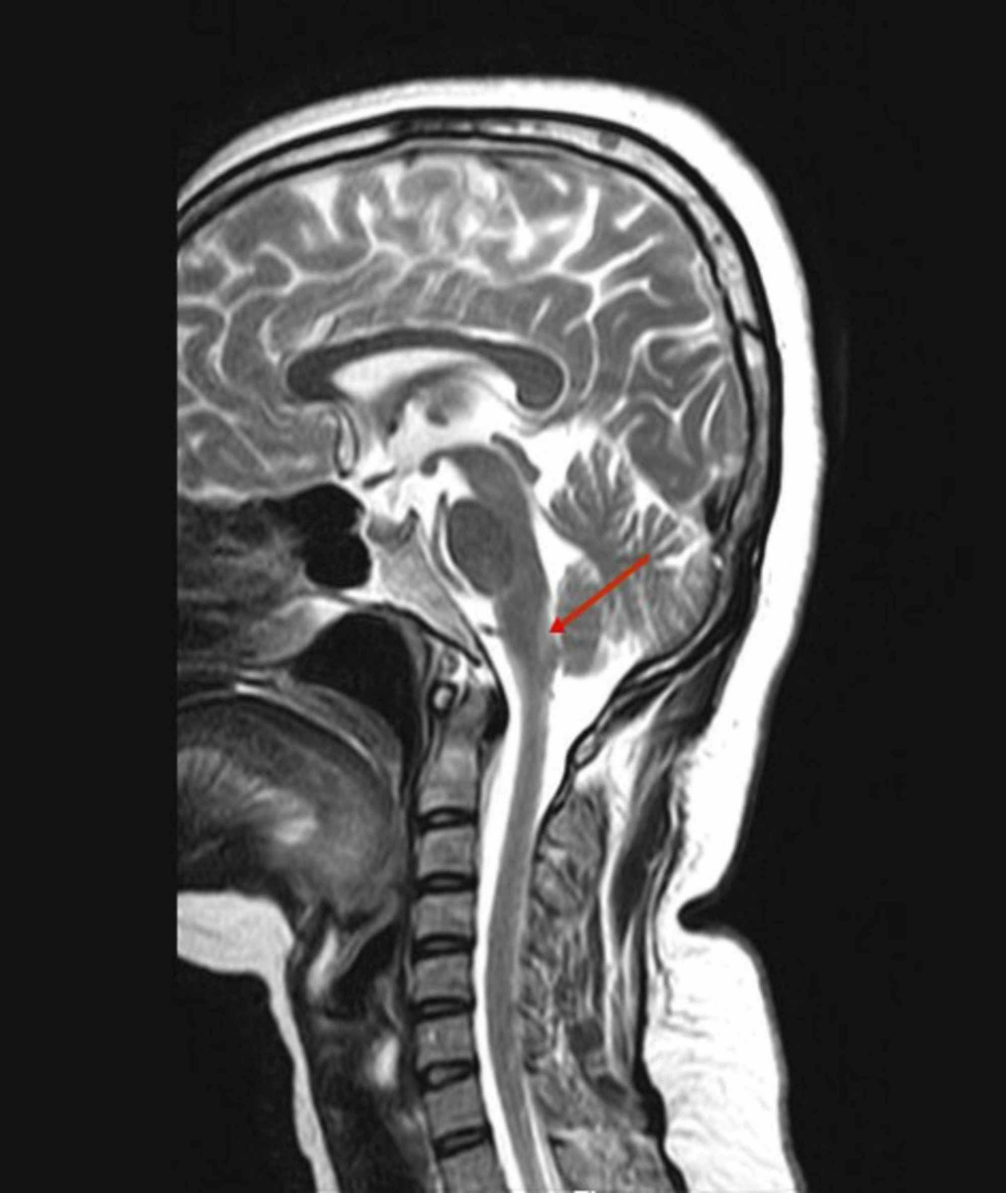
MRI spine showing an area of active demyelination in the periaqueductal region of dorsal medulla.
